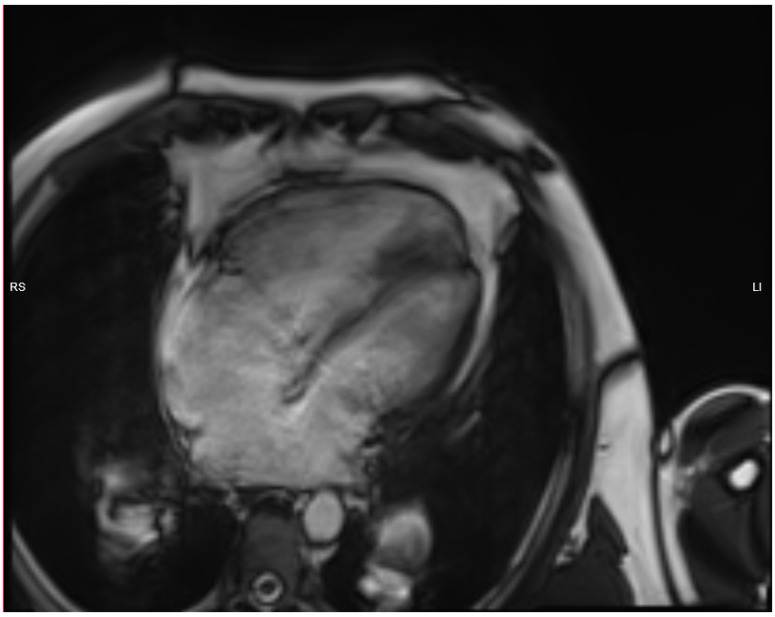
Magnetic resonance imaging shows the overload of the right heart and large secondary ASD (4.2 × 5.4 cm).
